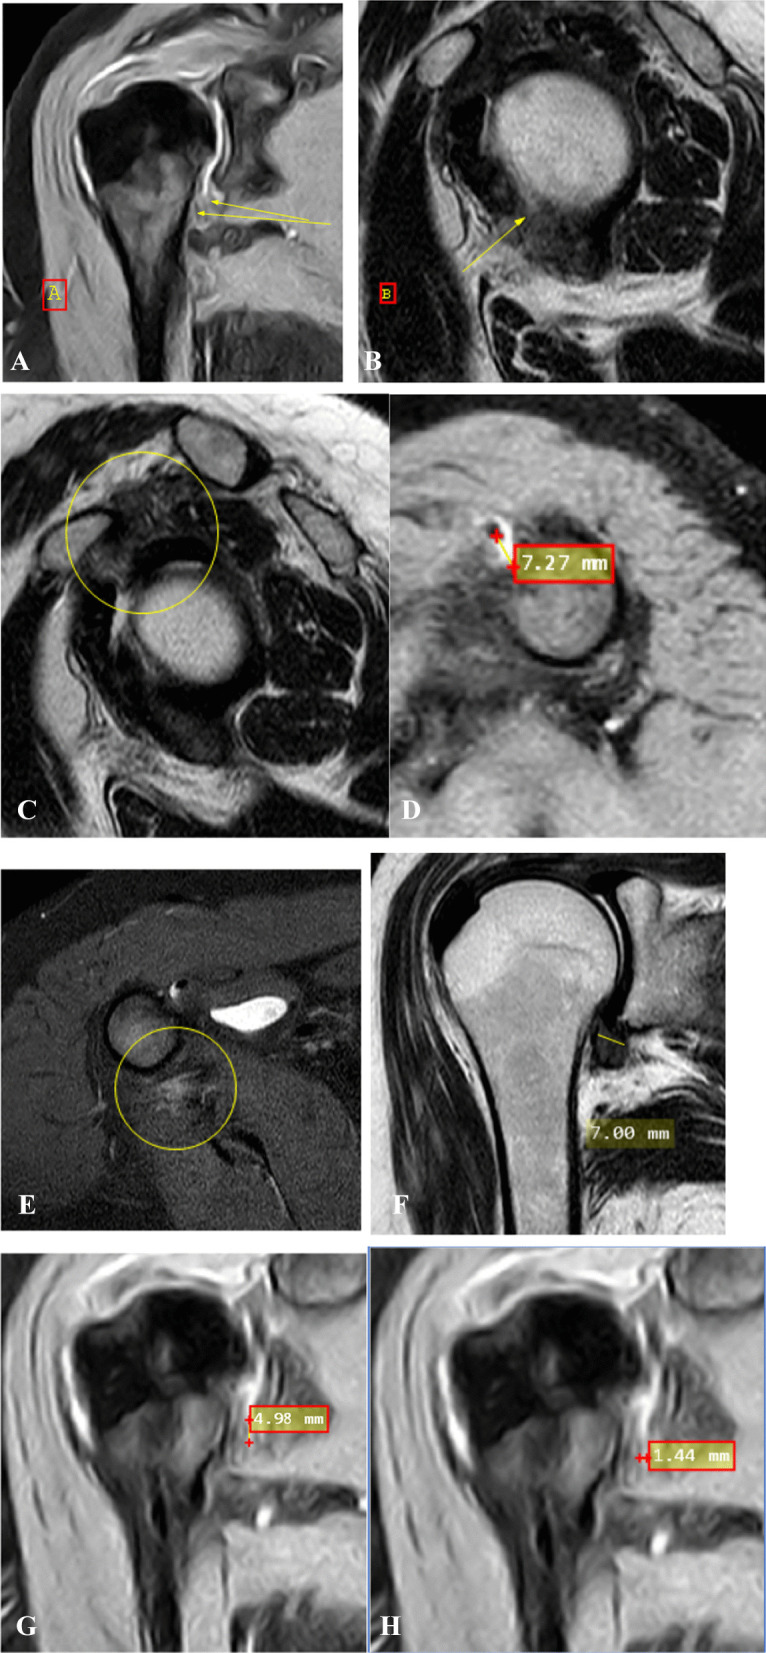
A Coronal fat-suppressed PD-weighted MRI image shows capsular edema at glenoid and humeral portions of capsule (arrows). B Sagittal T2-weighted MRI image shows anterior extracapsular edema (arrow). C Sagittal T2-weighted MRI image shows complete obliteration of subcoracoid fat triangle (encircled area). D Axial fat-suppressed PD MRI image shows effusion at long head biceps tendon sheath more than 2 mm (arrow). E Axial fat-suppressed PD-weighted MRI image shows extracapsular edema (encircled area). F Coronal T2-weighted MRI image shows maximum capsular thickening at glenoid portion of axillary recess, the humeral portion was obliterated and fibrotic. G, H Coronal fat-suppressed PD-weighted MRI image shows measurement of maximal height and width of axillary recess
